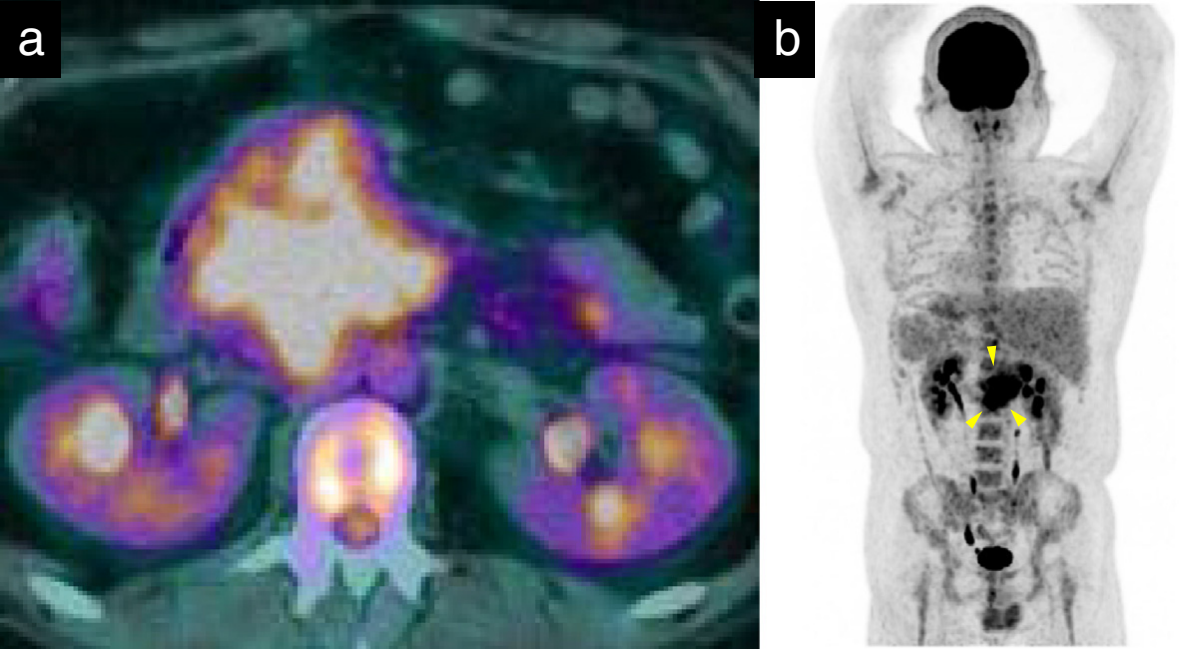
FDG-PET scanning of the abdomen and upper body. a The SUV max was 18.61. b There were no findings of metastases (*yellow arrows*)
